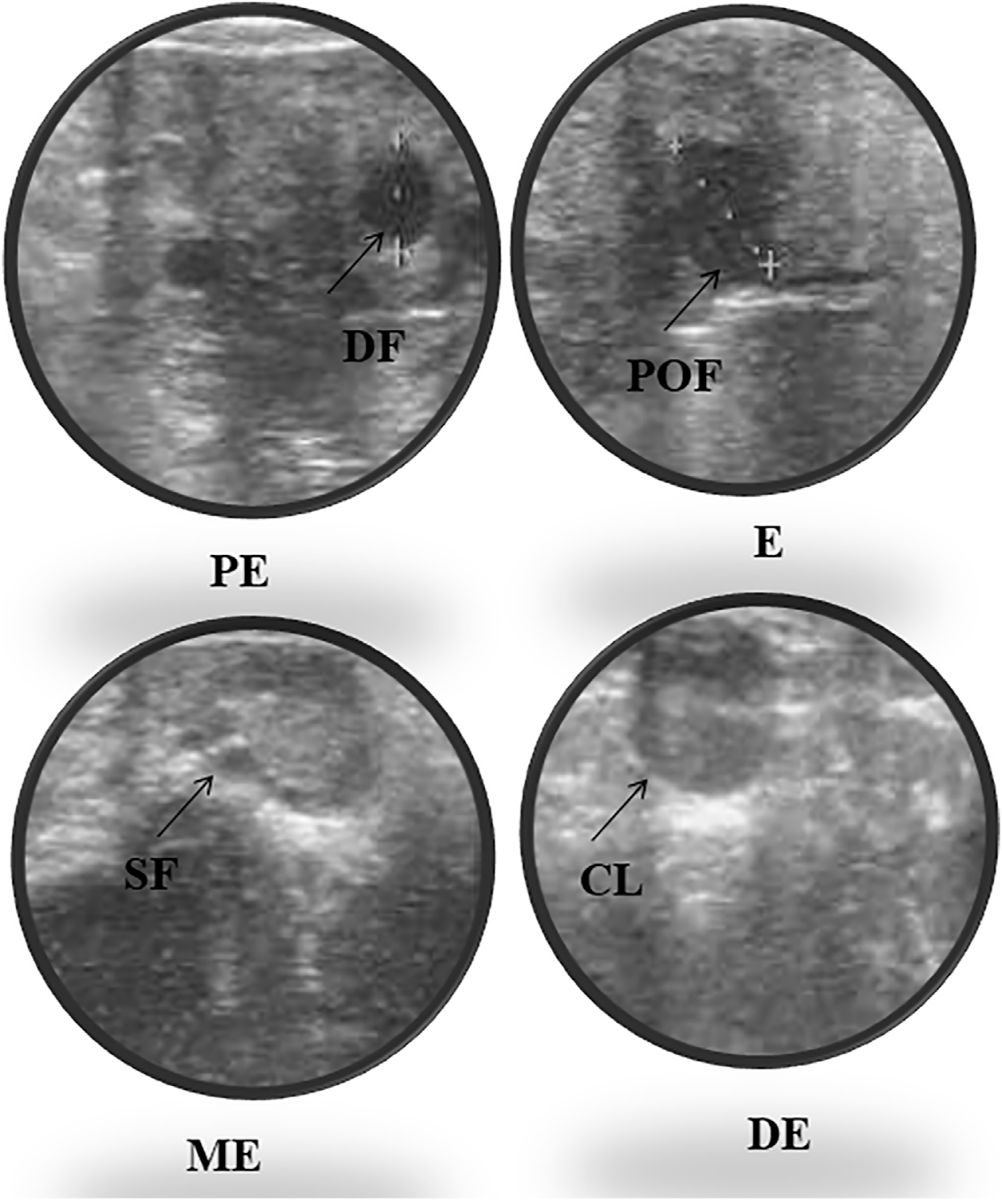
Ultrasonography of ovarian follicles and corpus luteum (CL) during proestrus (PE), estrus (E), metestrus (ME), and diestrus (DE) stages of the buffalo estrous cycle (DF, dominant follicle; SF, small follicle; POF, preovulatory follicle; CL, corpus luteum).
